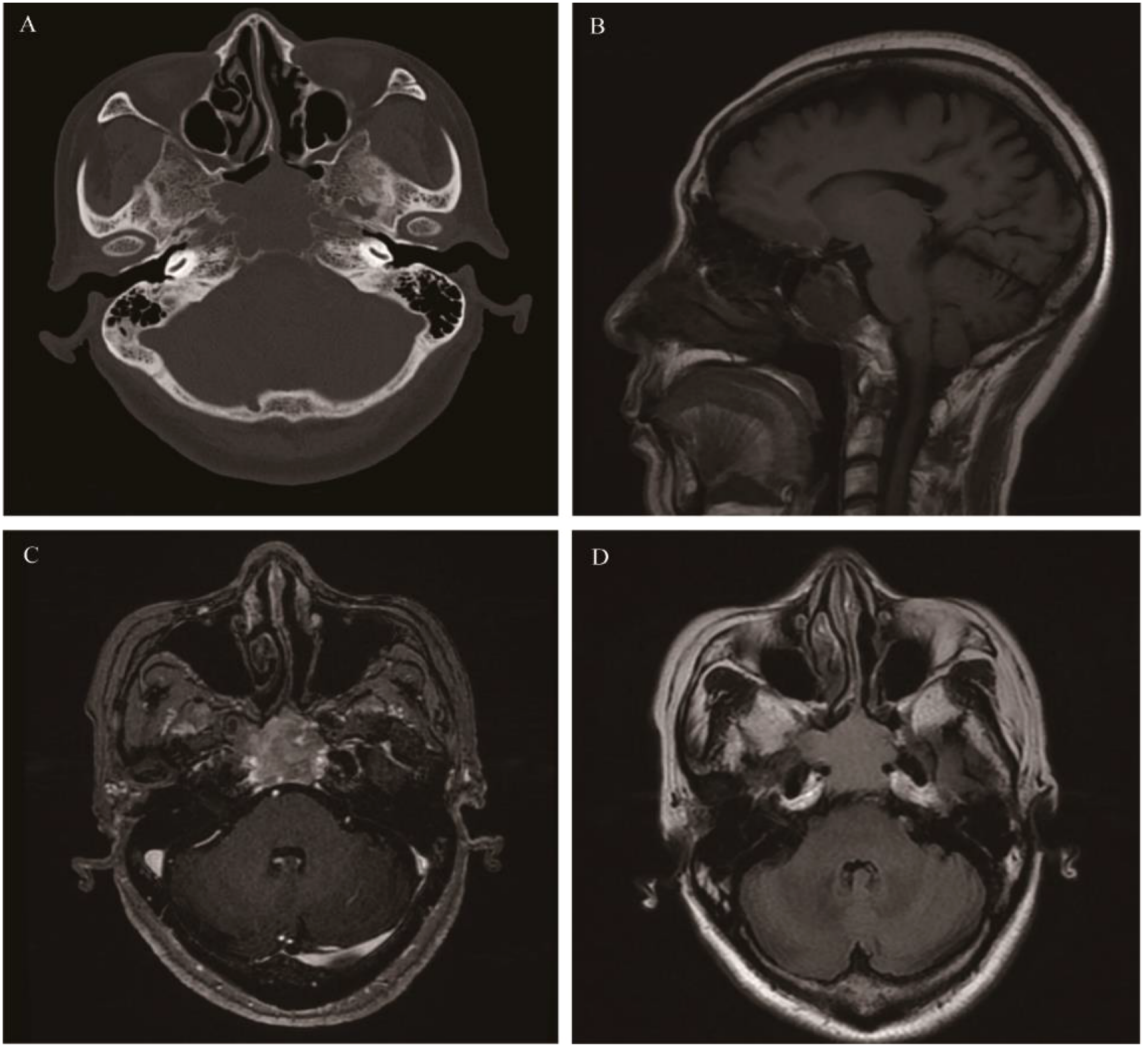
Ectopic pituitary adenomas: Axial CT showed an irregular soft tissue density mass involving the sphenoid sinus and clivus, with bone destruction mainly characterized by bone resorption and bone sclerosis formation (A). It appeared as isointense on T1WI (B), slightly hyperintense on T2 Fluid Attenuated Inversion Recovery (T2 FLAIR) (C), and showed significant enhancement on MR contrast-enhanced scan (D).
